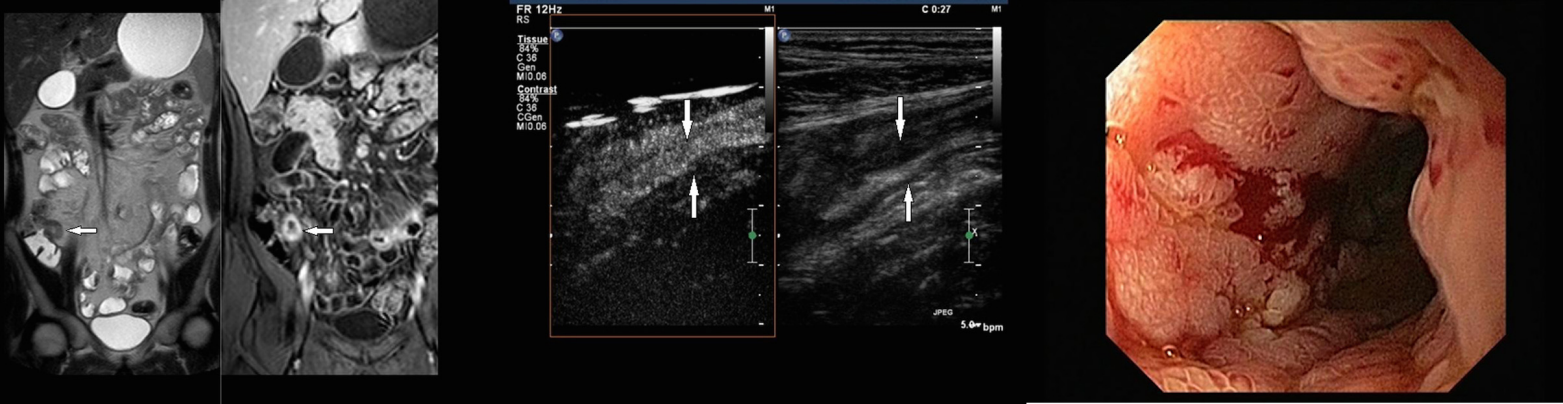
Twenty-five-year-old male patient with Crohn’s disease of the terminal ileum. A: Coronal MR Enterography images (T2-weighted single-shot turbo spin-echo on the left image; T1-weighted fat saturated, spectrally attenuated inversion recovery after Gadoteric acid administration, on the right image) of a patient with ileitis terminalis demonstrating marked wall thickening of the terminal ileum (white arrow) and mild enhancement after contrast administration (white arrow). B: Longitudinal Contrast enhanced ultrasound (Gray scale ultrasound image, on the right side; Contrast enhanced ultrasound image, on the left side), of the same patient showing wall thickening on Gray scale ultrasound (white arrow) and strong enhancement after Sonovue administration on Contrast enhanced ultrasound (white arrow). C: Endoscopic image of the same patient with an ulcerative ileitis terminalis.
